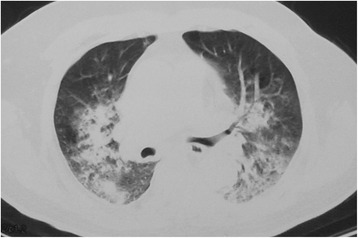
Radiologic finding in a 78-year-old cerebral infarct man with aspiration-related ARDS. Chest CT showed ground-glass opacities, inhomogenous patchy consolidations and pleural effusion in bilateral lobes
